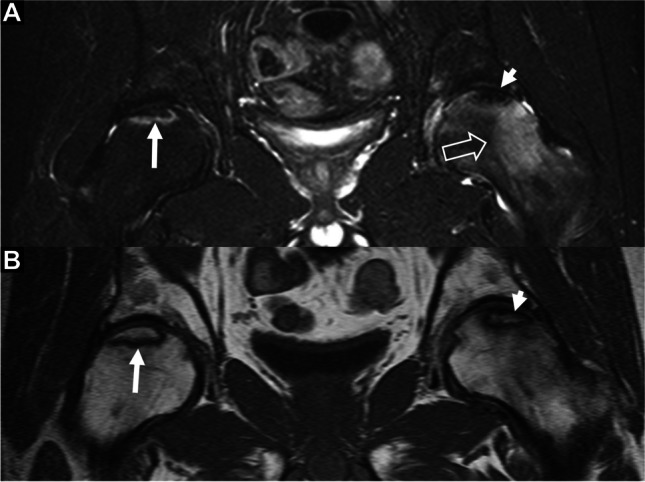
Coronal STIR (A) and T1-w (B) MR images, showing bilateral idiopathic avascular necrosis, in a 43-year-old male presenting with a left painful hip. The lesion on the right hip (arrows), is asymptomatic and is occult on plain radiographs (not shown). The lesion on the left is associated with bone marrow edema (open arrow) secondary to mild articular surface flattening (short arrows). According to ARCO classification, the right lesion is stage I and the left stage III
